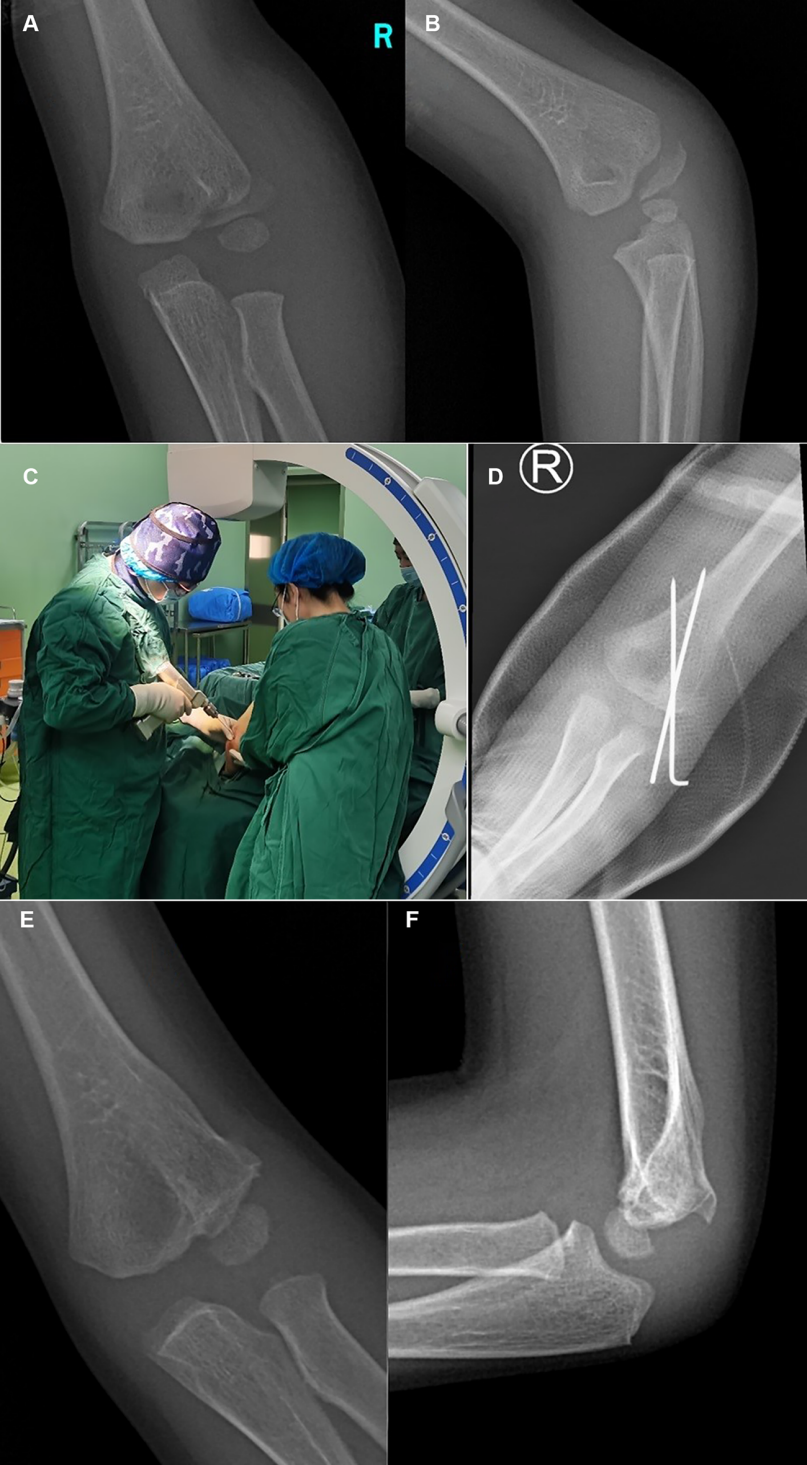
Typical x-ray of Type I fractures. Anteroposterior (AP) (A) radiographic image of the injured elbow, showing a Type I fracture with displacement of >2 mm. Internal oblique (B) radiographic image of the injured elbow, displaying a Type I fracture with even more obvious fracture fragment displacement. The position (C) of the assisting surgeon and operating surgeon during manipulation. (D) AP radiograph of the right elbow that was treated with two different 1.6 mm K-wires in CRPP. Typical X-ray of type I. AP (E) and lateral (F) radiographic images showing fracture union at 10 months postoperatively.
